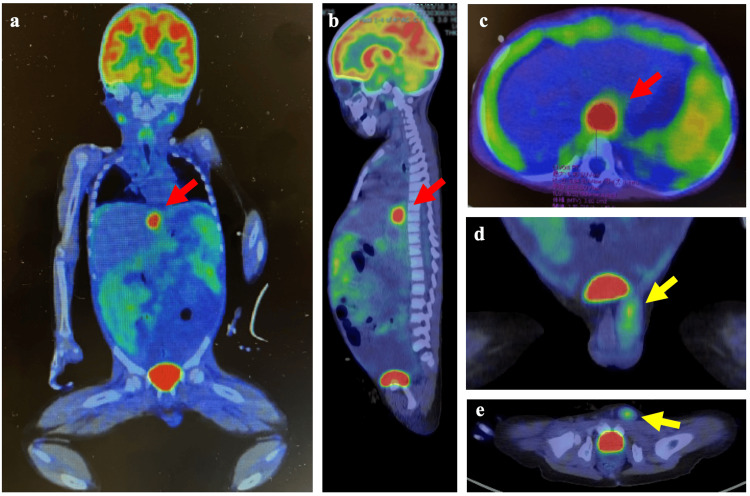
2-deoxy-2-(fluorine-18)-fluoro-D-glucose positron emission tomography/computed tomographic images. The red arrows indicate the primary tumor lesion on a coronal image (a), a sagittal image (b), and a horizontal image (c). The yellow arrow indicates a metastatic lesion extending from the left inguinal canal into the scrotum on a coronal image (d) and on a horizontal image (e). The volume of the neoplastic lesion originating from the diaphragm was 3.60 m³, and that of the neoplastic lesion in the left inguinal region was 0.41 m³.
